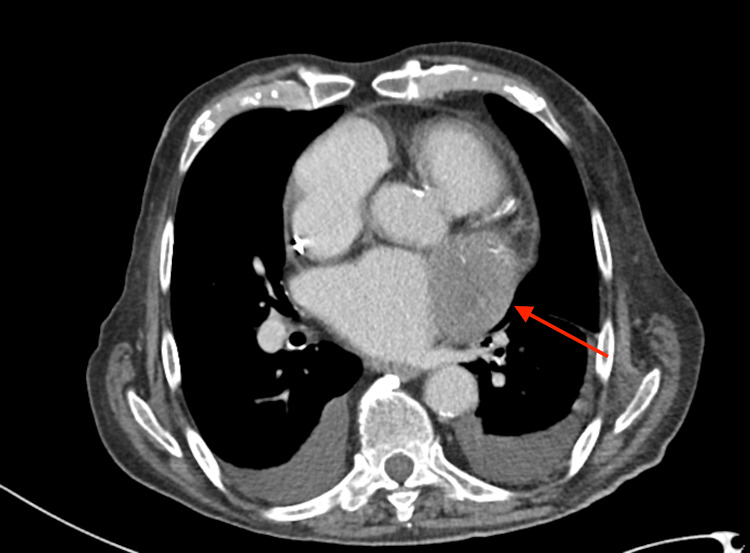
Axial section of CT-thorax demonstrating hypodensity at the level of the left auricle
